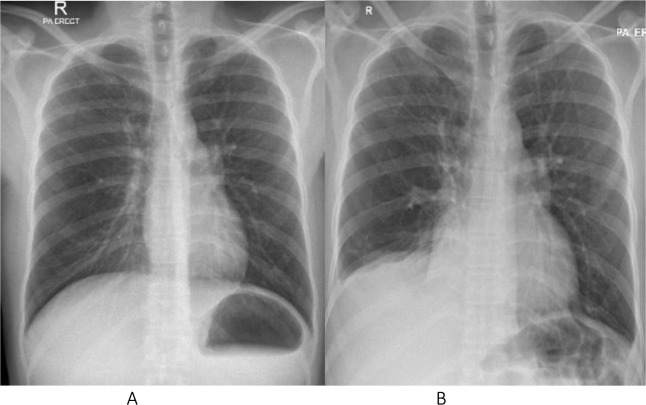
(A) Chest X-ray showed clear costophrenic angles compared to (B) – the image on admission, which showed blunting in the right costophrenic angle.
